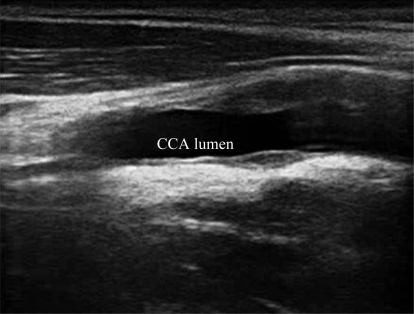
A sub-image from a B-mode sonographic image sequence. There are plaques on both near and far walls. (Sequence S1: Male, 36 yr old).
